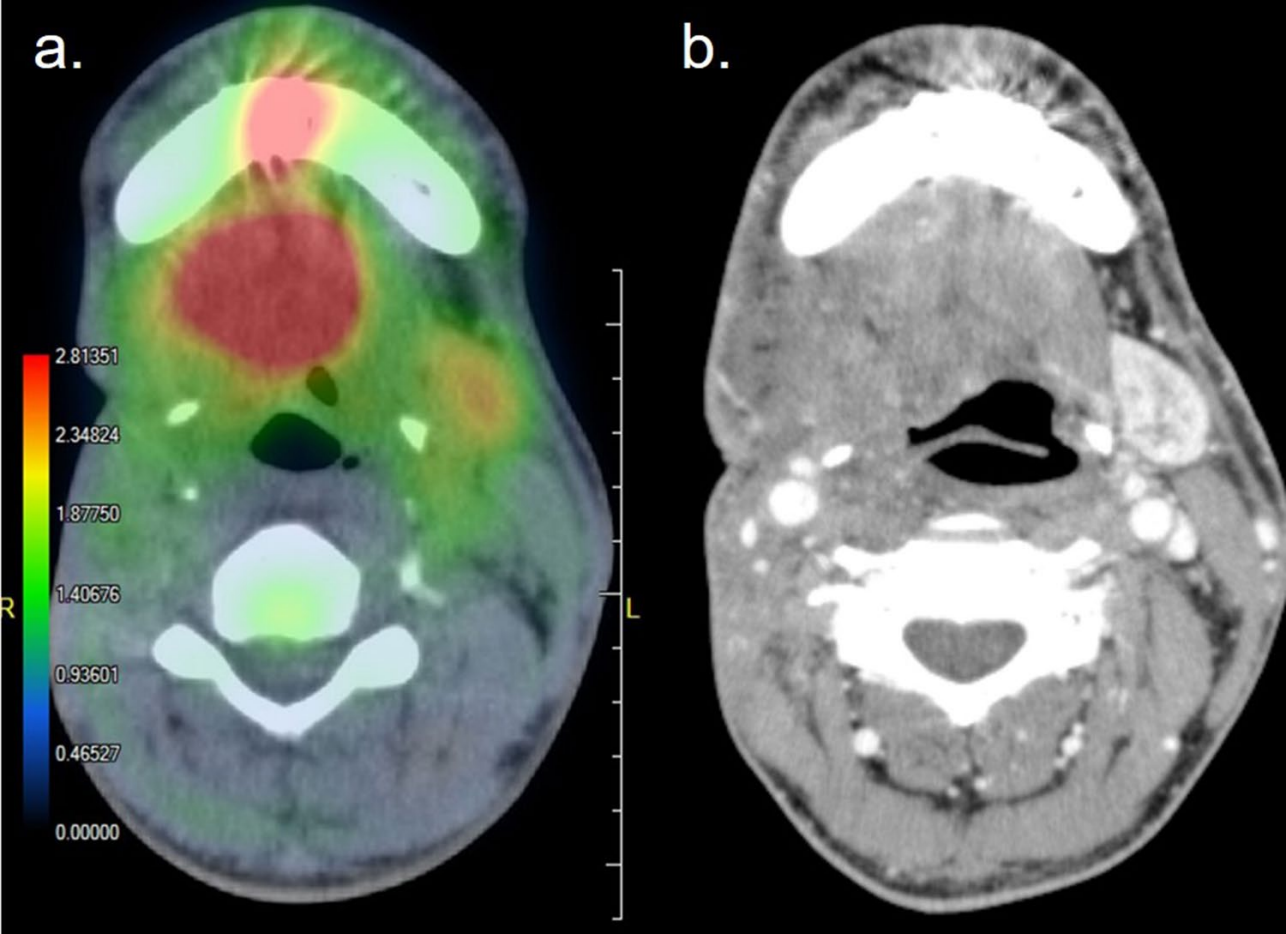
Patient with post-operative-state tongue cancer. An intense FDG accumulation seen in the center of the reconstructed tongue (a) without any abnormality on the contrast enhanced CT (b) or clinical inspection. The interview revealed that he had underwent rehabilitation for postoperatively impaired oral muscular function before the PET examination
